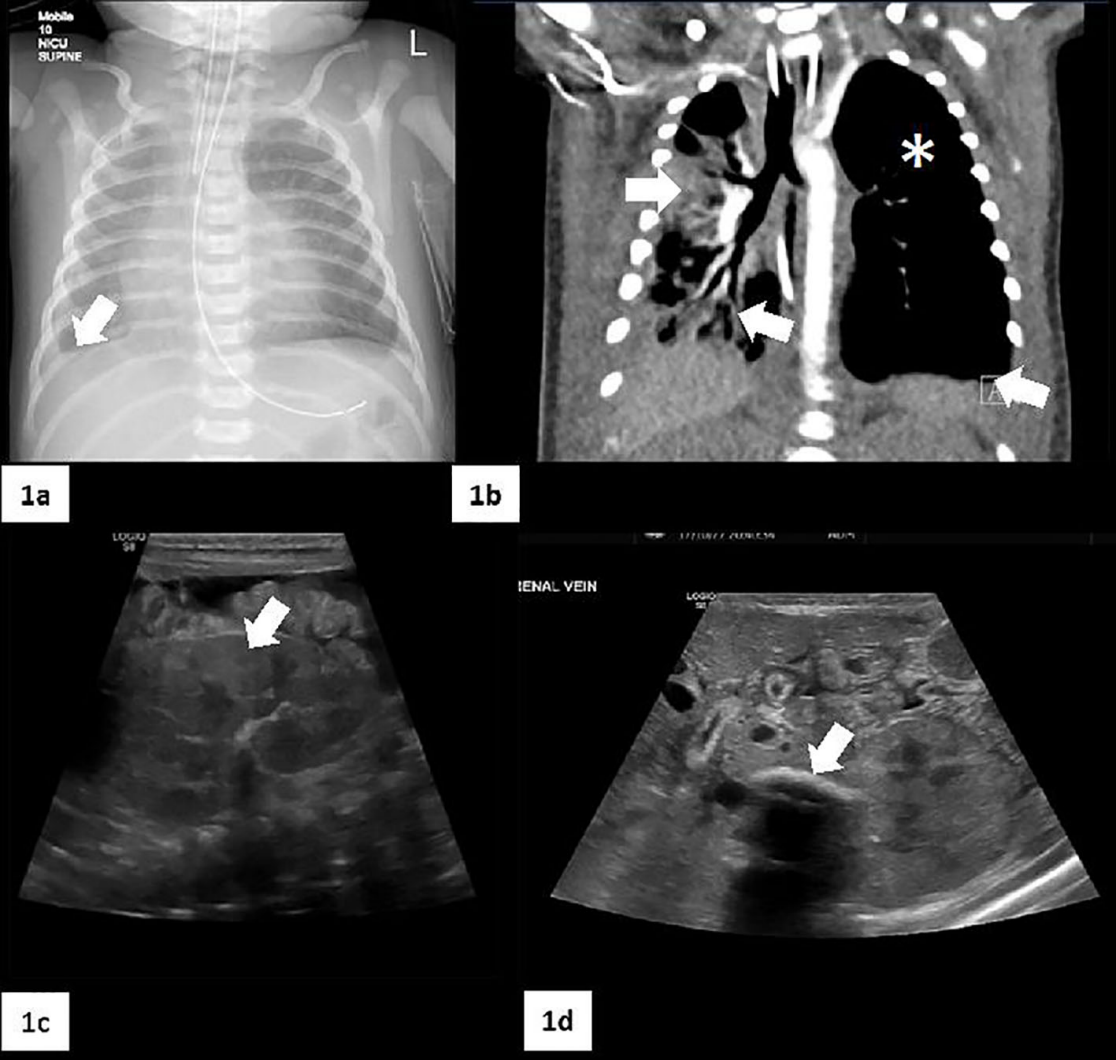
Radiological imaging. (A) Chest X-Ray that shows a moderate right pleural fluid collection (white arrow) accompanied by a volume loss in the right lung. (B) CT scan: small right lung with a predominantly central/perihilar consolidation with air bronchograms (two white arrows). The left lung is slightly hyperexpanded, with no interstitial changes and small areas of atelectasis (white asterisk). There are small bilateral pleural effusions (white arrow). (C) Marked abnormality of the kidneys with cortical echogenicity (white arrow). (D) Shows a calcified left renal vein thrombosis (white arrow). Free fluid in the peritoneum with some internal strands suggestive of chronicity.
